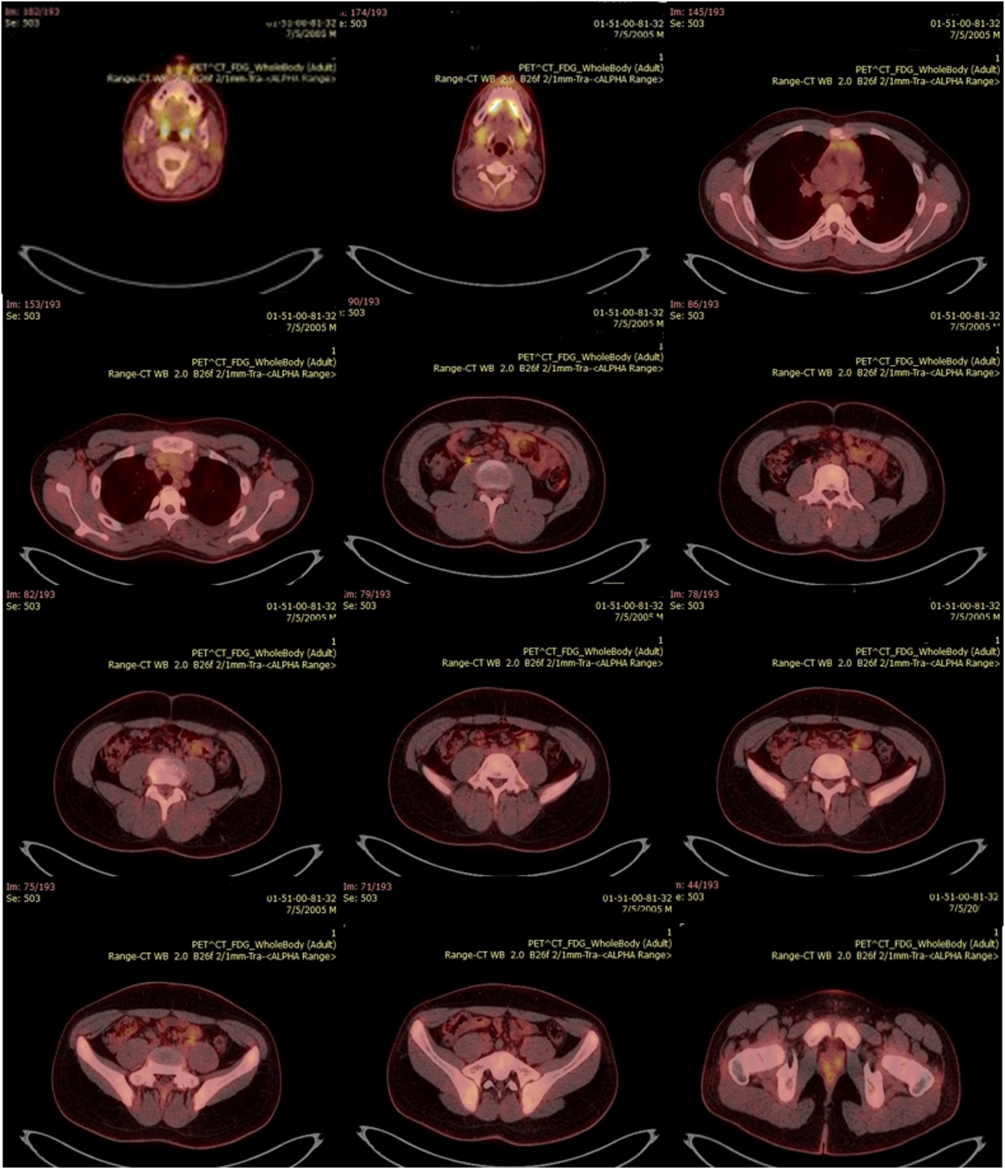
PET/CT image. The PET/CT result indicated no sign of lymph proliferative disorders in the patient
